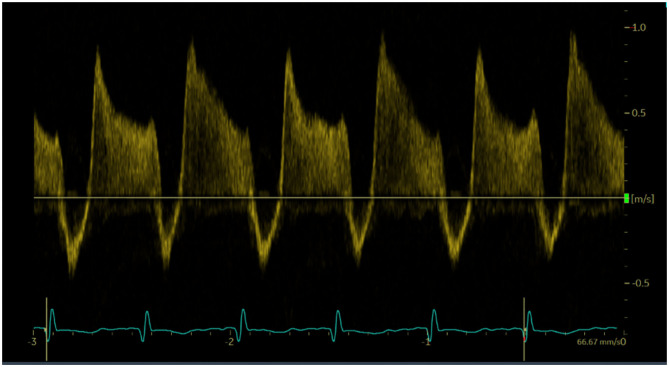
Doppler pulse wave measurements of ductus arteriosus flow. Three consecutive flows profiles were used to average measurements.
